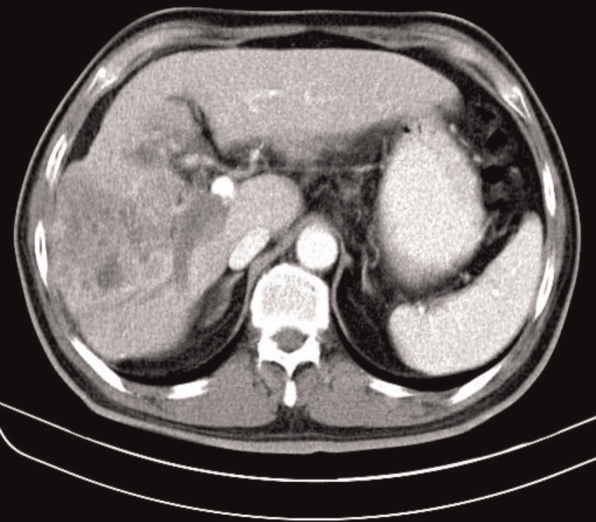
Computed tomography scan of the abdomen shows a large liver mass with heterogeneous enhancement in the right hepatic lobe.
